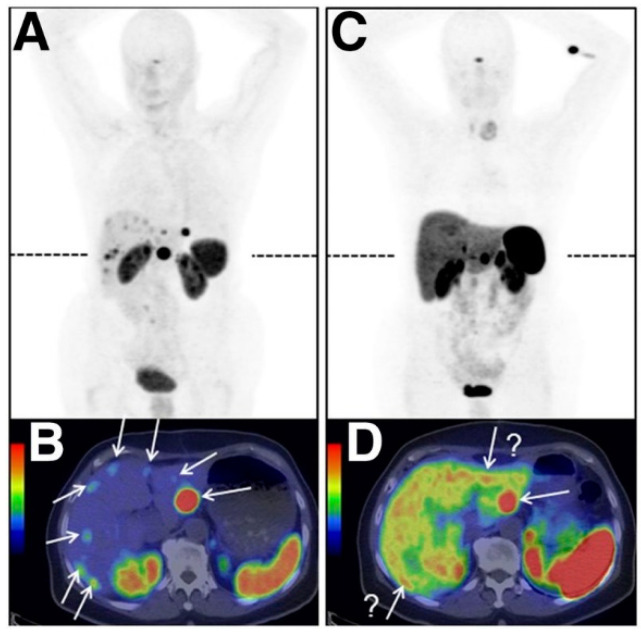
PET/CT images of a patient with ileal neuroendocrine tumors showing bilobar liver metastases (marked with arrows) after application of [^68^Ga]Ga-OPS202 (A) and its transaxial fusion image (B) and [^68^Ga]Ga-DOTATOC (C) and its transaxial fusion image (D) (adapted from [60]).
